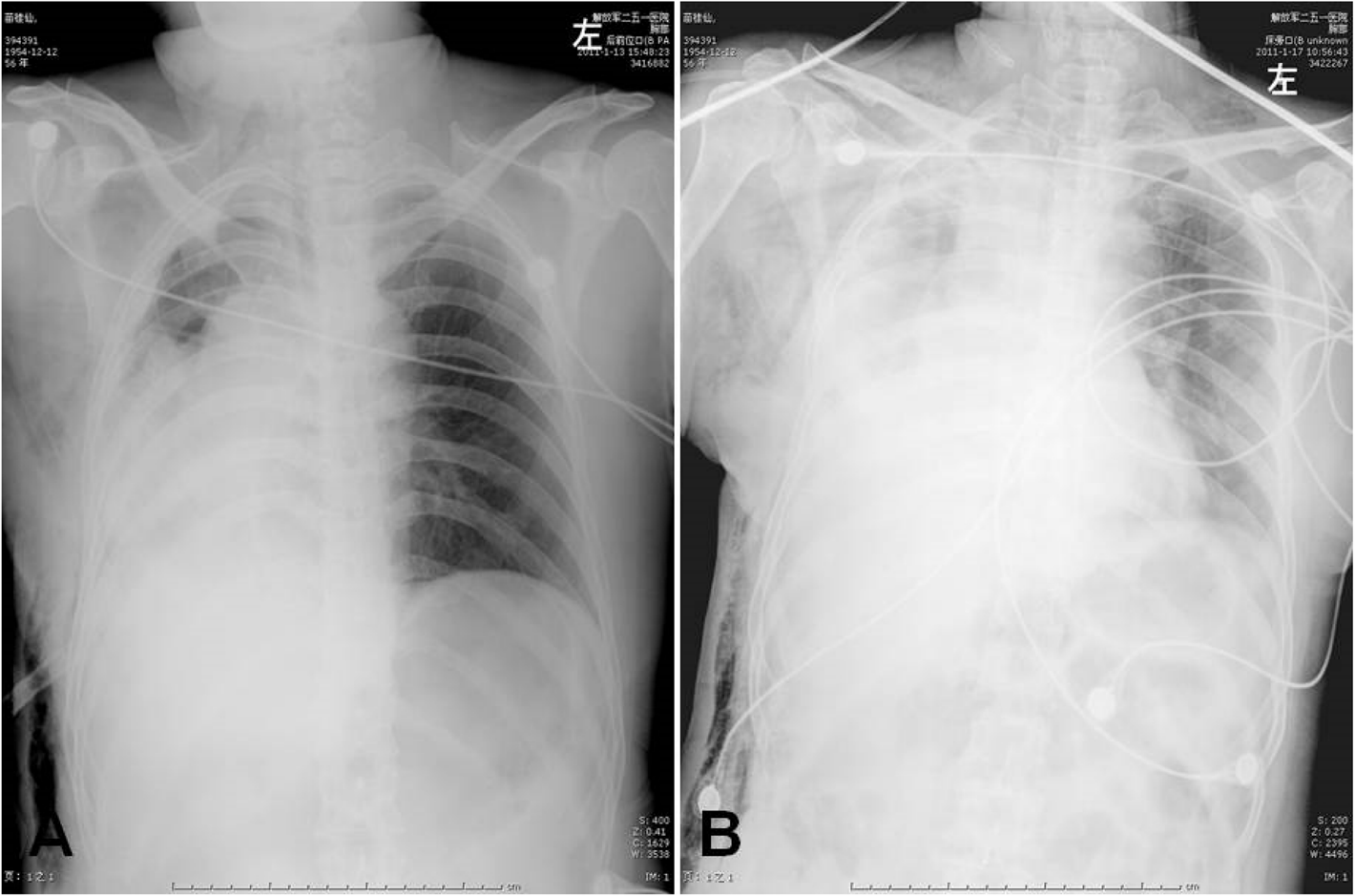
Chest radiography showed a shift of the heart into right hemithorax after 18 h of intrapericardial pneumonectomy (a), and the apex of heart returned into normal position after rethoracotomy (b)
